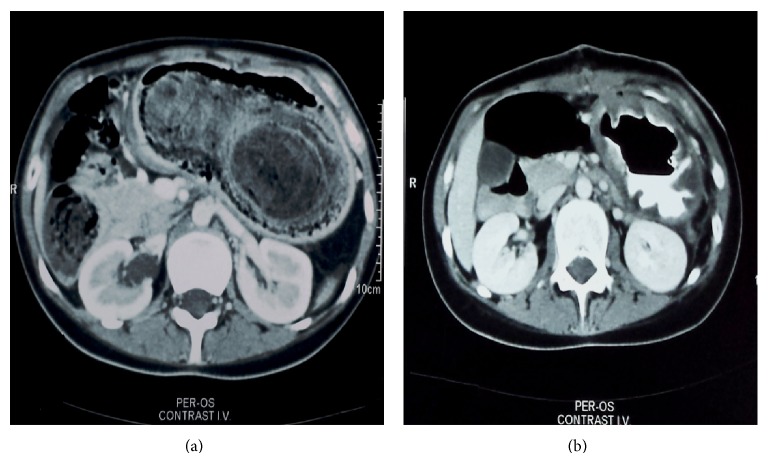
CT scan of the abdomen before the operation revealing a giant trichobezoar in the stomach and extending to the duodenum (a) and a CT scan of the abdomen 5 days after the operation showed massive dilation of the stomach (b).
